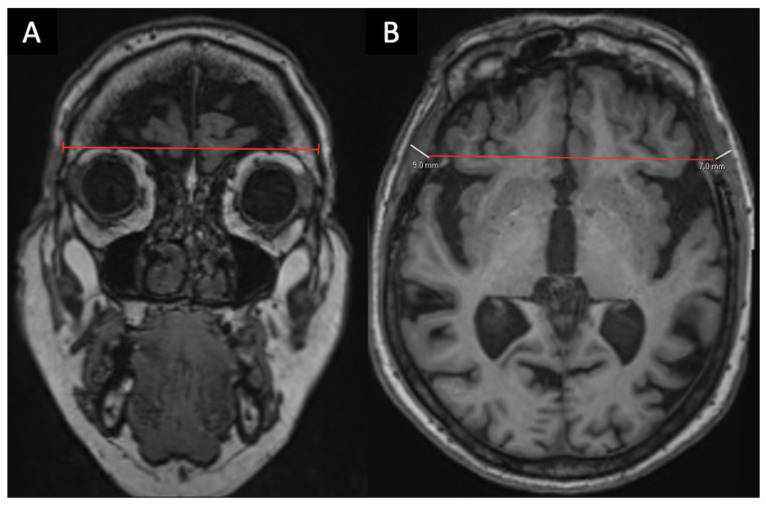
Measurement of Temporal Muscle Thickness (TMT). In order to ensure reproducibility, measurements of TMT (mm) are performed on axial T1-weighted sequences in the axial plane (B) using a simple measurement tool on the PACS system, as the maximum perpendicular distance between the outer table of the cranium and the inner layer of the superficial temporal fascia, typically at the level of the superior orbital roof, found in the coronal plane (A).
